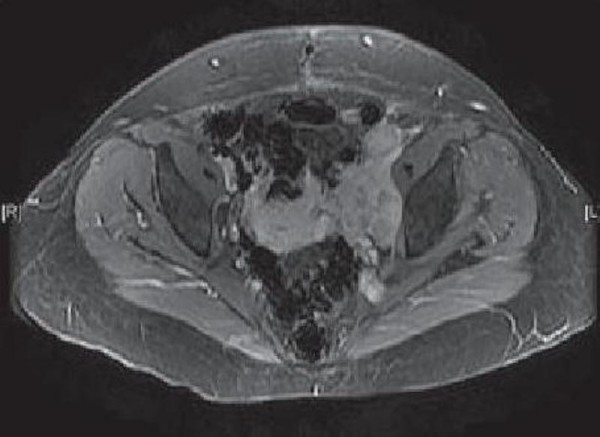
MRI showing the extent of disease before therapy: Cross-sectional view of lobulated left pelvic mass measuring 7.8 × 6.6 cm.
